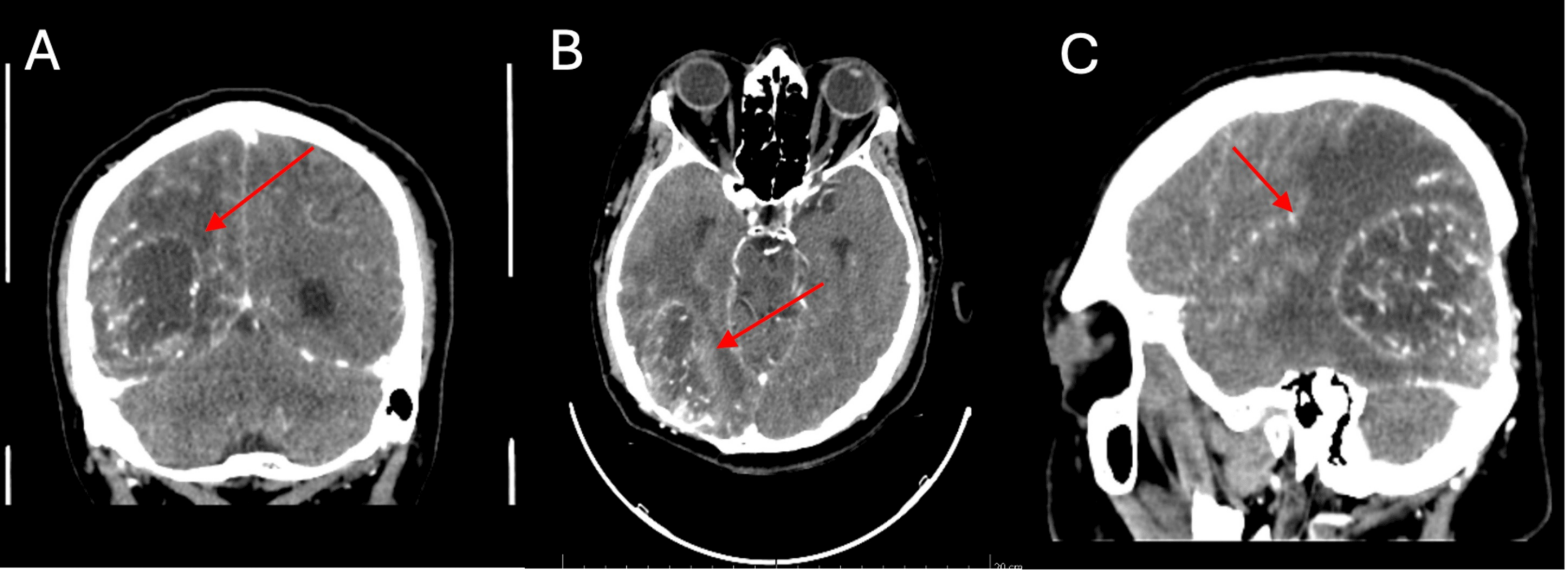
Brain CT scan with the presence of a heterogeneous space-occupying lesion, right parietooccipital, with extensive perilesional edema, multiple hypointense vascular structures inside, with moderate enhancement, irregular contours. A. Coronal section. B. Axial view. C. Sagittal view
